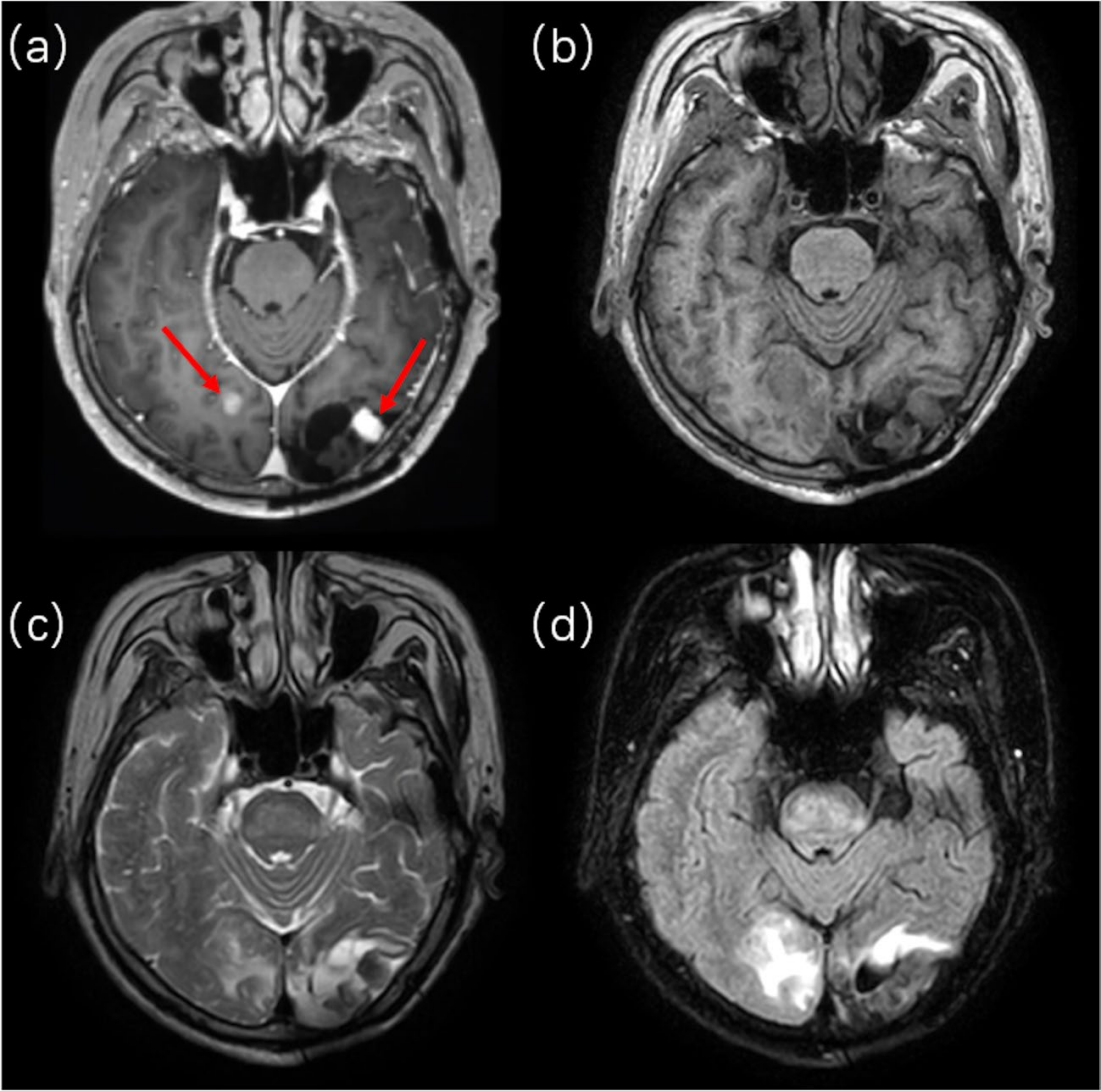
Two lesions were clearly visible on the right and left hemispheres, respectively, on (a) pre-treatment T1c image but difficult to locate in the images of (b) online-T1, (c) online-T2, and (d) online-FLAIR
